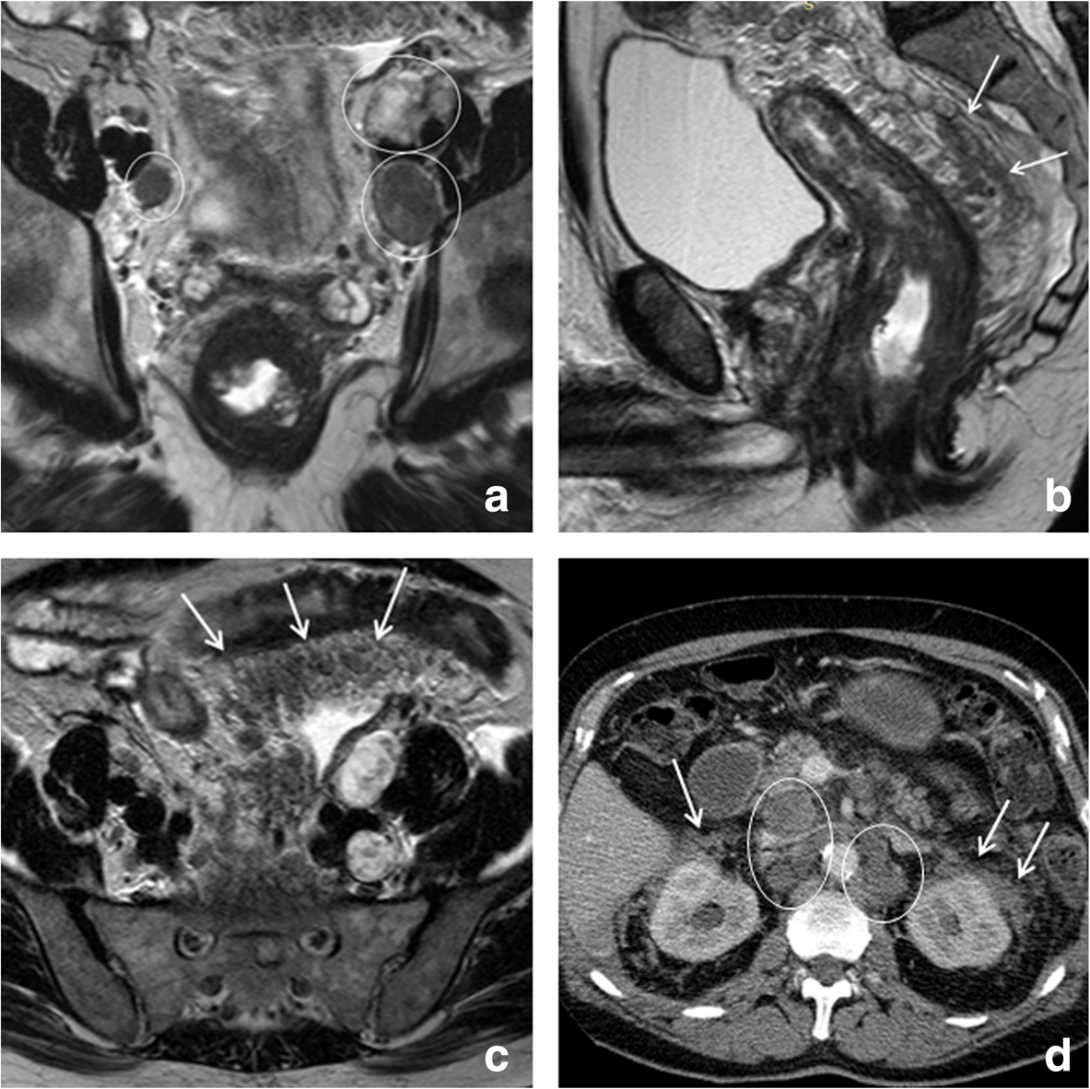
Signet-ring tumor. Transverse (a), sagittal (b) and coronal (c) T2-weighted MR images, and CT image (d) of a male patient with a signet-ring carcinoma of the rectum. Note the typical diffuse hypointense thickening of the rectal wall with extensive tumor infiltration in the mesorectal fat (arrows in b), that extends all the way up cranially into the mesosigmoidal fat (arrows in c). There are several large metastatic lymph nodes both in the pelvis (circles in a) as well as para-aortic in the upper abdomen (circles in d). At the time of diagnosis, the patient presented with extensive peritoneal metastases (arrows in d). Palliative chemotherapy was started, shortly after which the patient deceased
